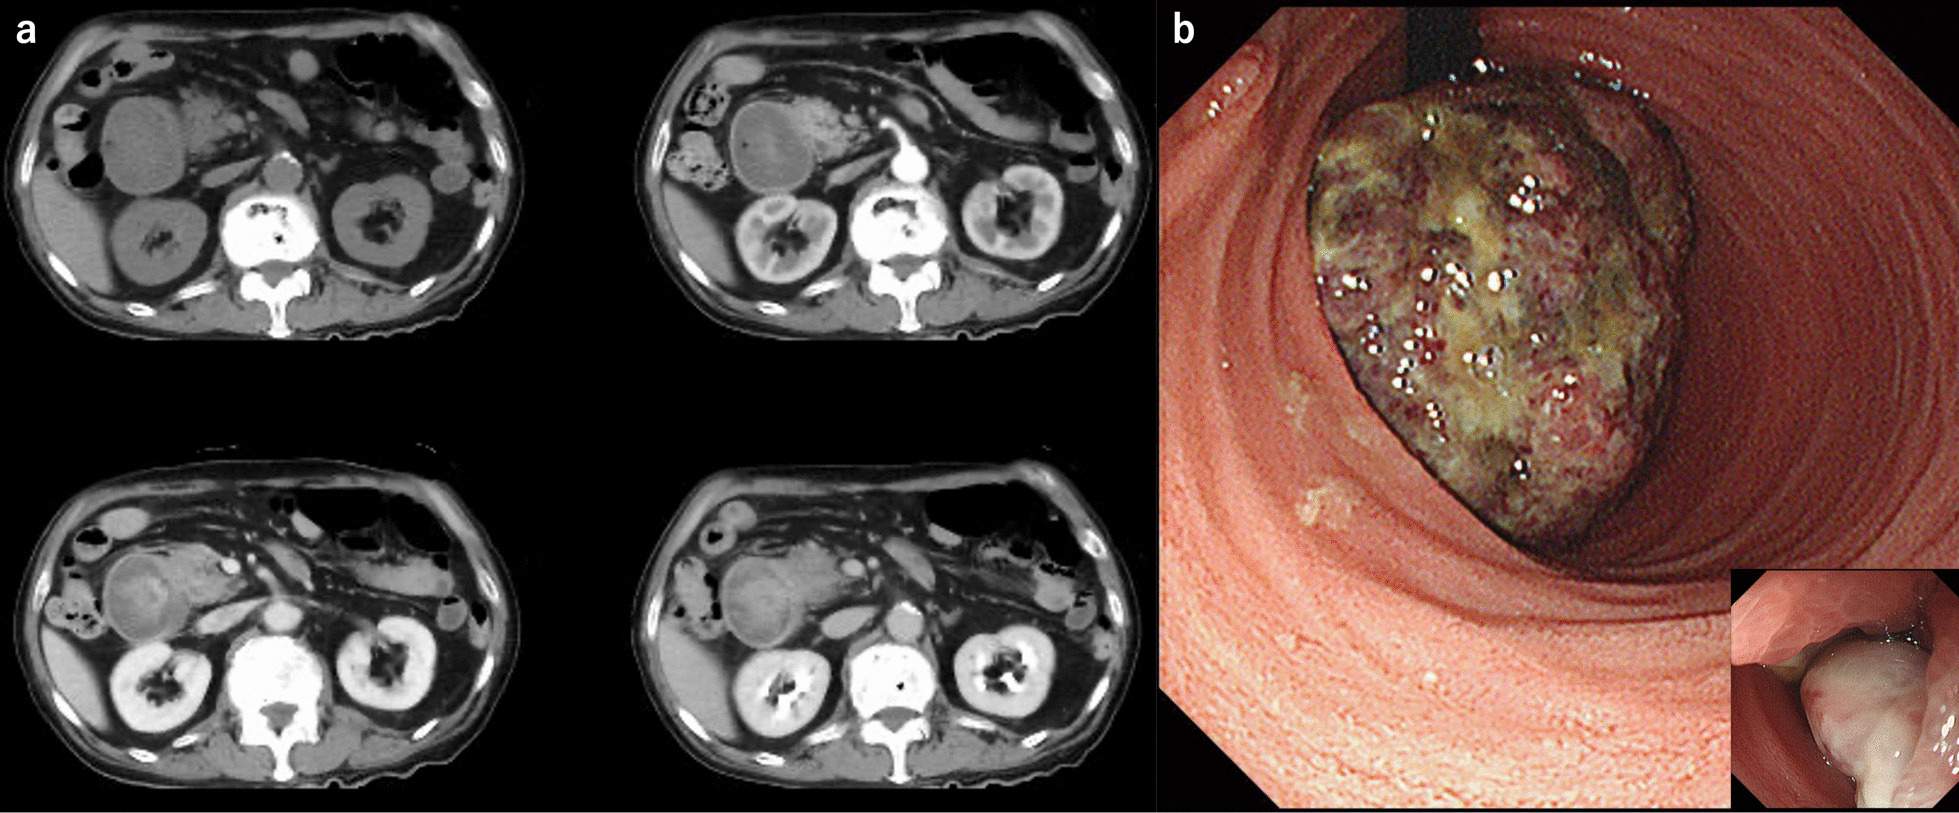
a Abdominal contrast computed tomography findings. Gastric outlet obstruction and duodenal wall thickening. b Upper gastrointestinal endoscopy findings. A type 1 tumor arising from the gastric antrum and extending into the duodenal bulb
